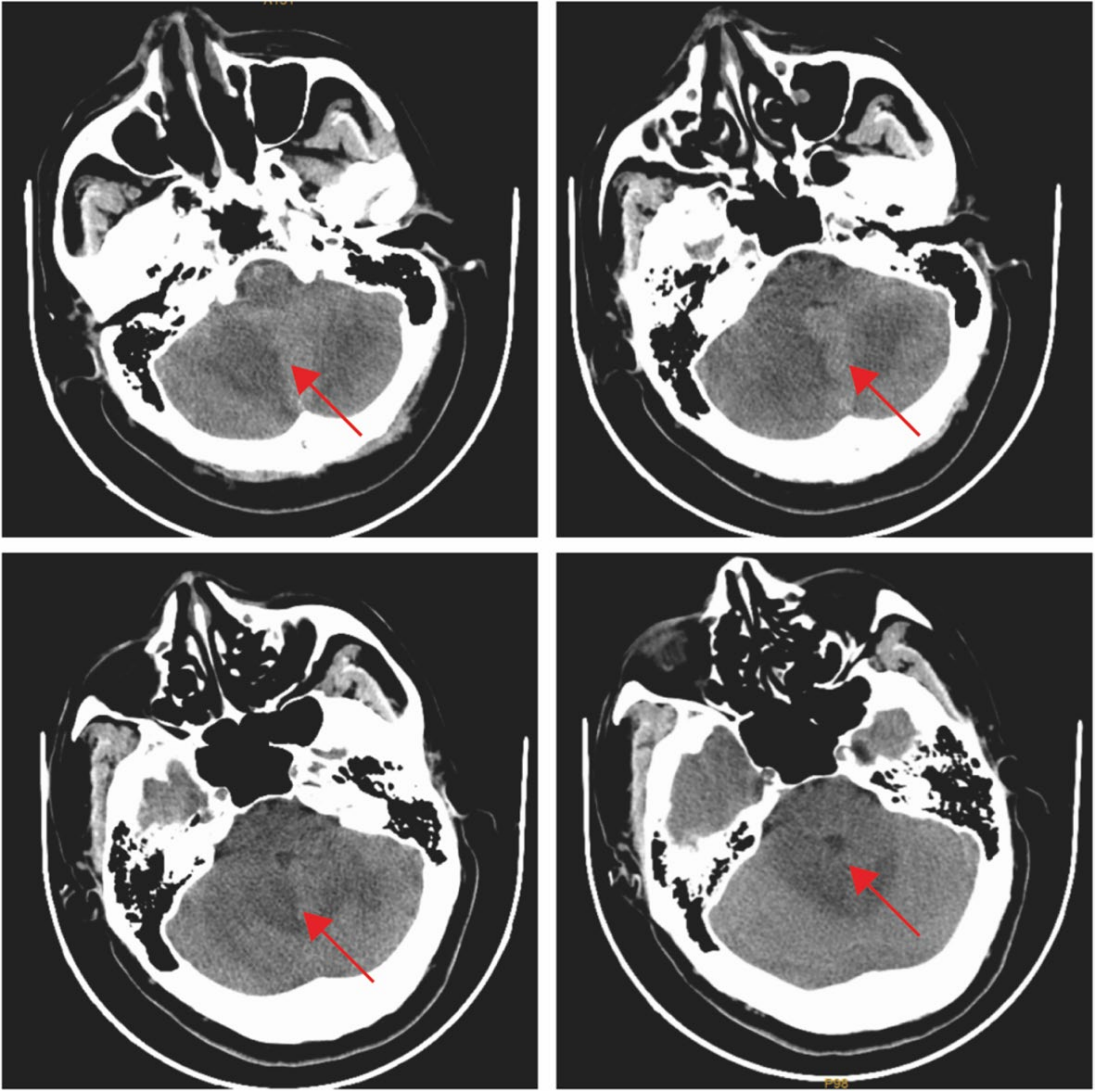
Plain head CT: bilateral cerebellar lesions. Arrowheads: infection lesions
